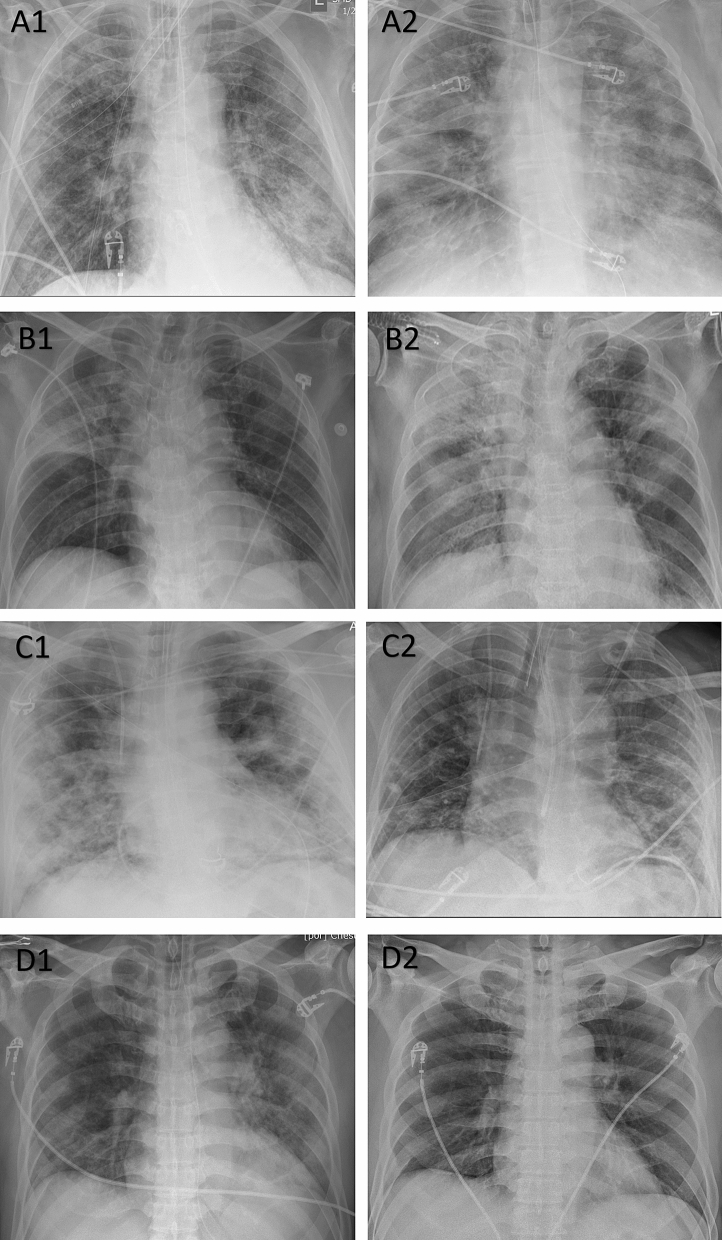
Frontal CXRs of patients with RT-PCR positive COVID-19 pneumonia. A A 65-year-old male from Site A with baseline (A1: RALE score 12, AI score 101) and follow-up CXRs (A2: RALE score 32, AI score 175) received mechanical ventilation and died after 30 days of hospitalization. B An 84-year-old male from Site B with baseline (B1: RALE score 10, AI score 59) and follow-up CXRs (B2: RALE score of 29, AI score 127) received mechanical ventilation and expired after 17 days of hospitalization. C A 58-year-old male from Site A had full recovery following hospitalization (baseline CXR, C1: RALE score 24, AI score 156; follow-up CXR, C2: RALE score 9, AI score 133). The patient required mechanical ventilation. D A 57-year-old male from Site B had full recovery following hospitalization. The radiographic opacities on baseline CXR (D1: RALE score 11, AI score 98) resolved on follow-up CXR (D2: RALE score 0, AI score 0). The patient did not require mechanical ventilation.
